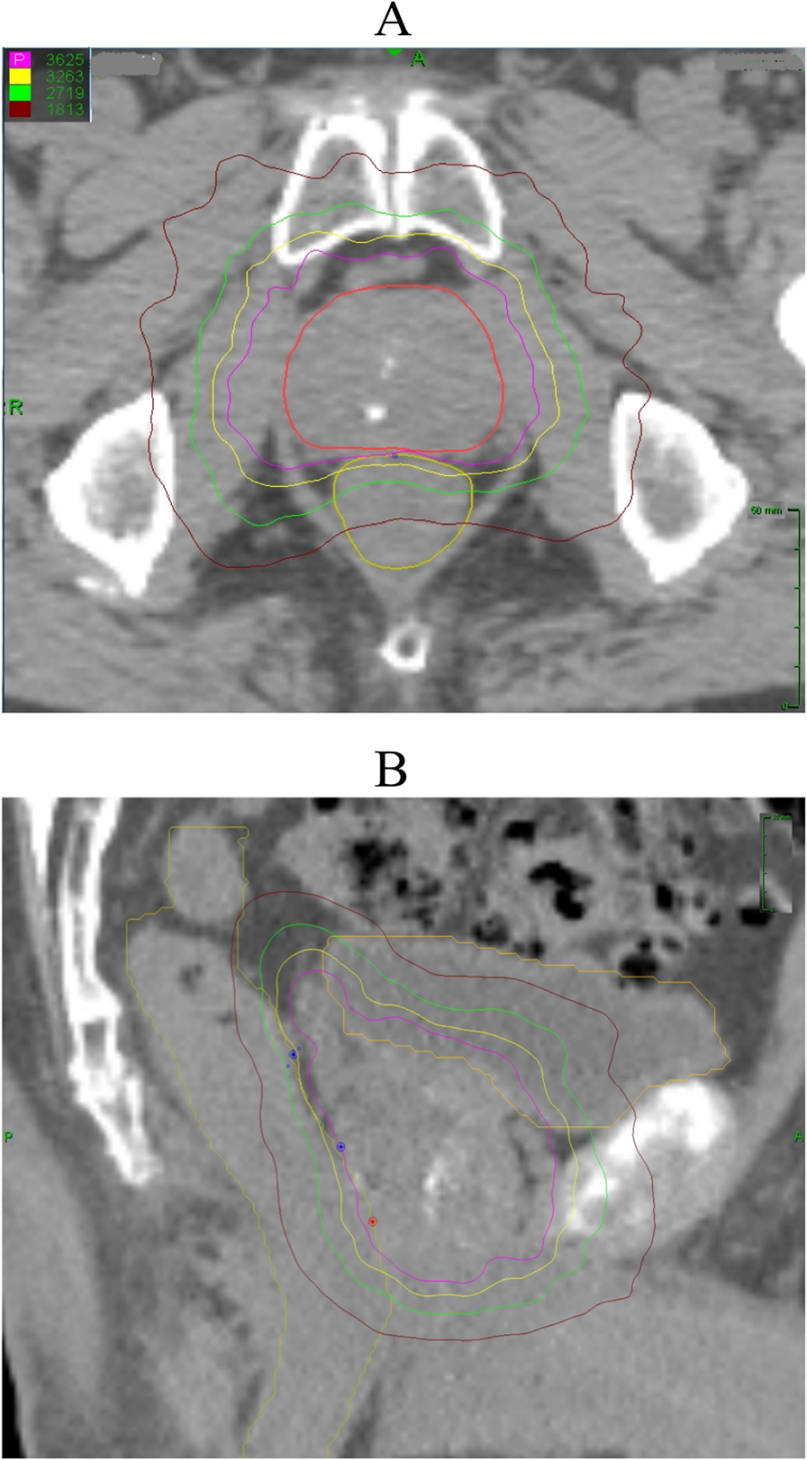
Treatment planning scan. Axial (A) and sagittal (B) computed tomography images the prostate GTV (red line), bladder (orange line) and rectum (yellow demonstrating line). Isodose lines shown as follows: 100% of the prescription dose (lavender line); 90% of the prescription dose (yellow line); 75% of the prescription dose (green line); 50% of the prescription dose (brown line).
